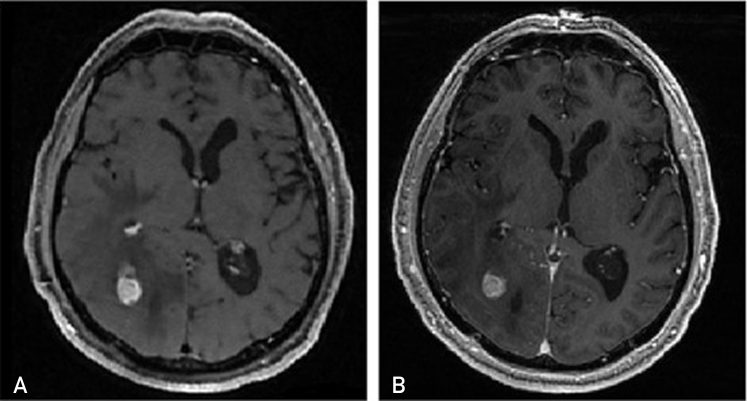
(A) A tumor with contrast-enhancing lesions using a 3-dimensional fast spin echo magnetic resonance imaging (MRI). (B) The same tumor and time point using an inversion recovery gradient echo MRI.
